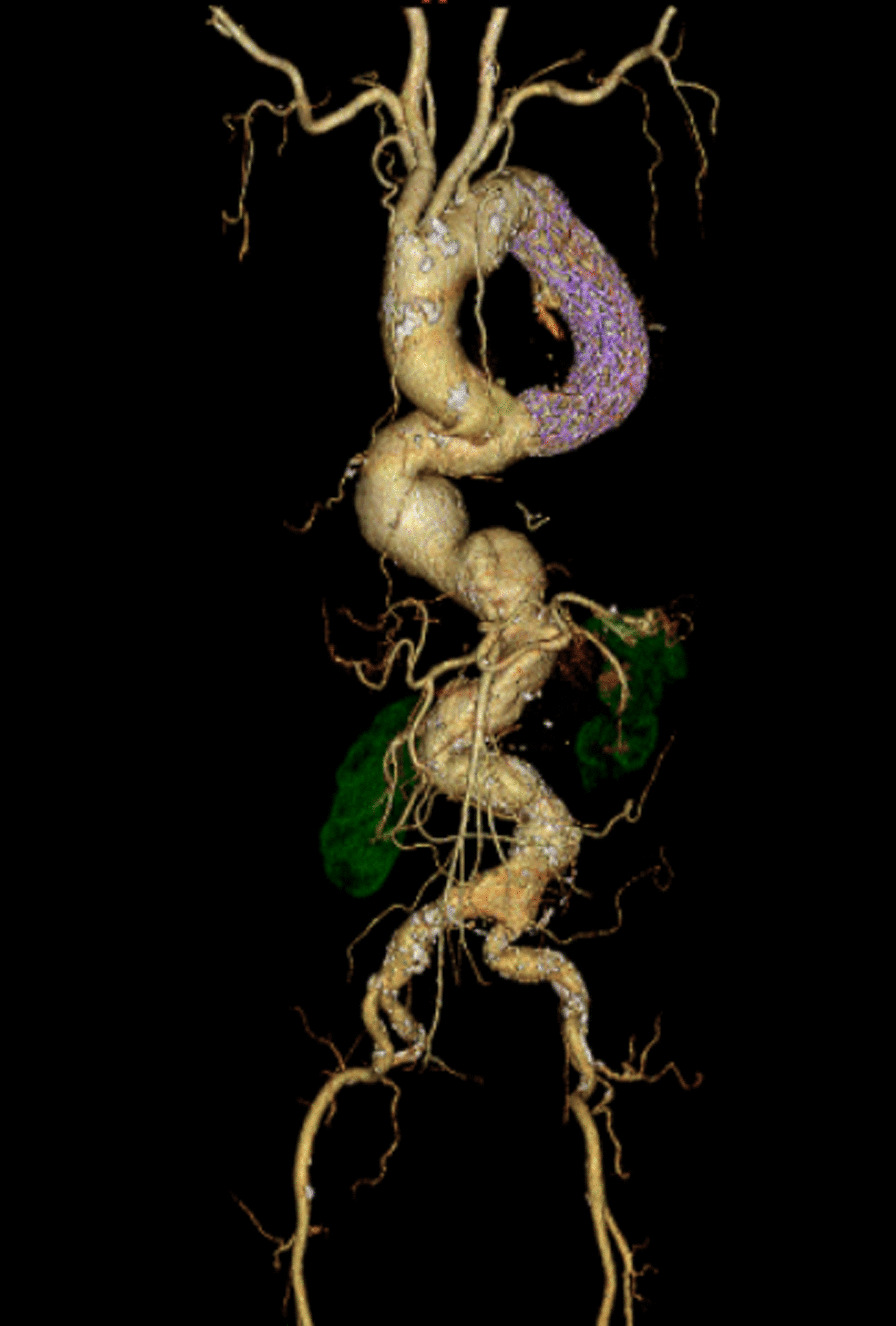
Postoperative computed tomography image. Postoperative contrast-enhanced CT images showed no endoleak
